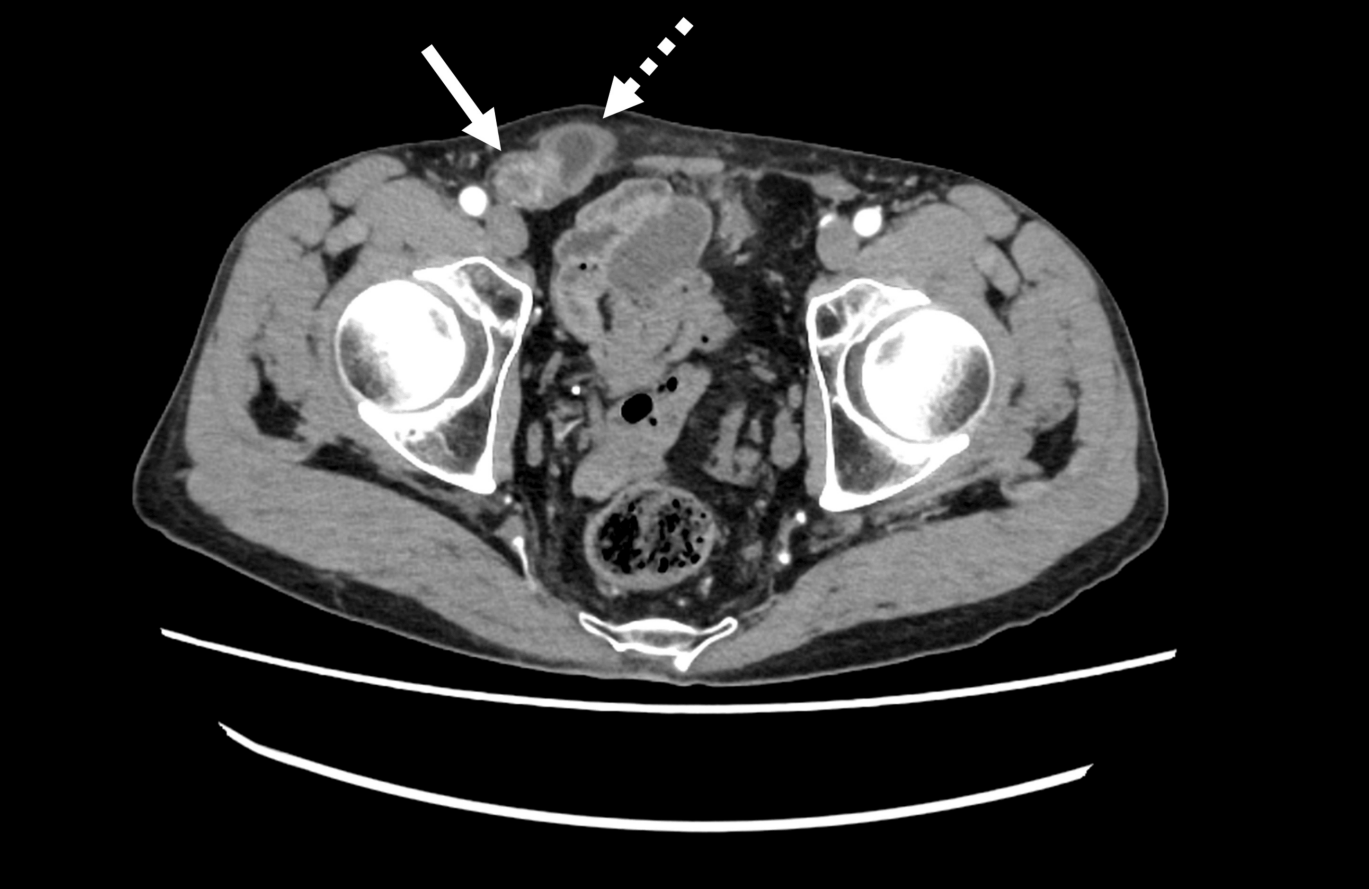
Abdominal contrast-enhanced computed tomography Axial contrast-enhanced computed tomography shows a spermatic cord mass (dotted white arrow) in the right inguinal region, along with herniated small bowel and fatty tissue (white arrow), indicating an incarcerated inguinal hernia.
